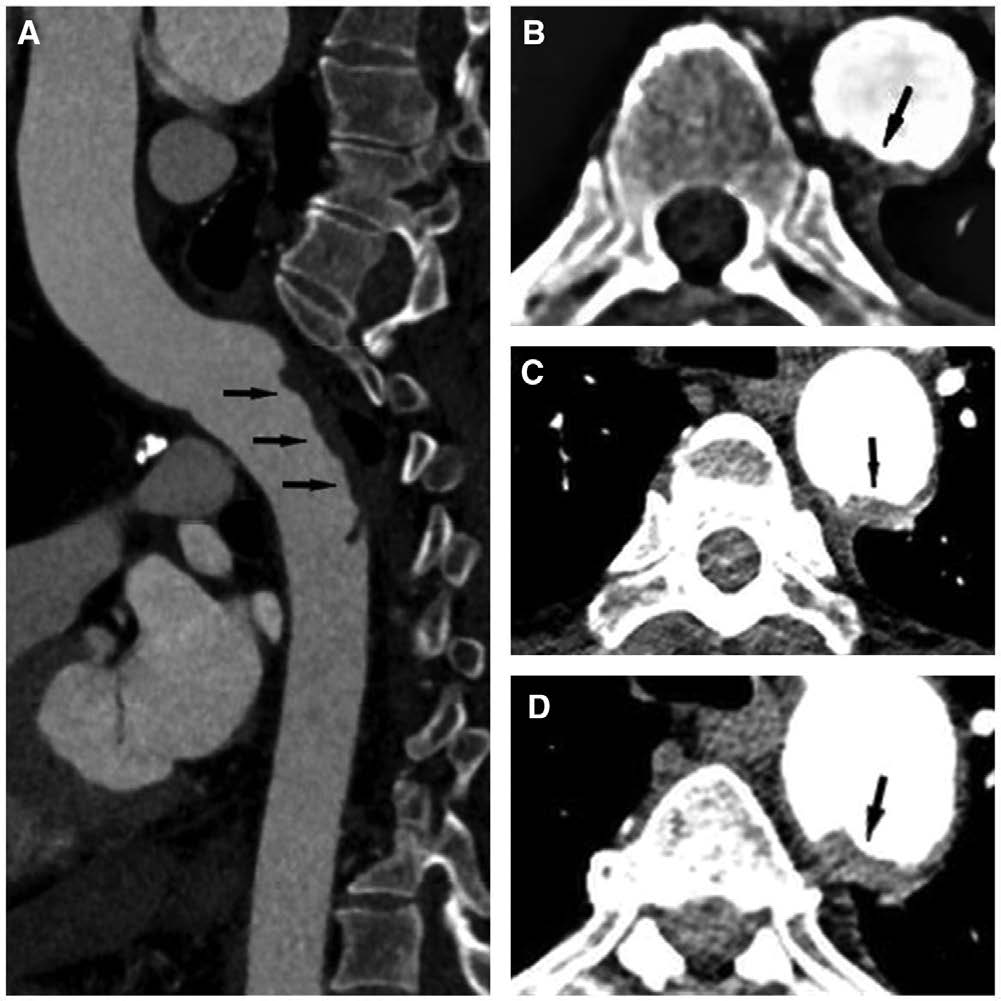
CTA of thoracic aorta on sagittal (A) and axial (B–D) views displayed descending aorta thrombosis at the level of thoracic vertebral bodies from T4 to T6 (black arrow). CTA = computed tomography angiography.
